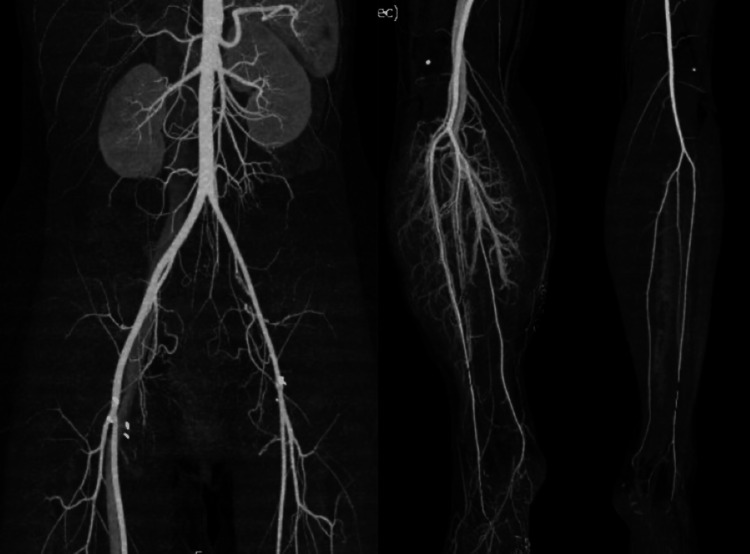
Post-operative contrast-enhanced CT The flow to the limbs was improved. CT, computed tomography
